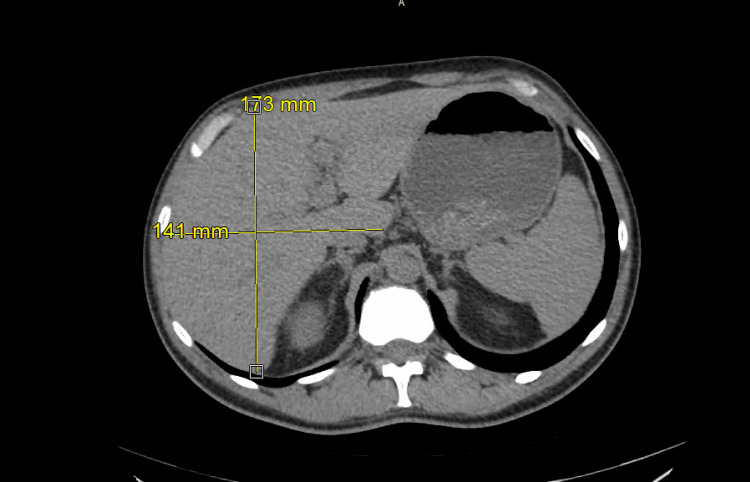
The CT abdomen shows hepatomegaly measuring 173 mm x 141 mm
